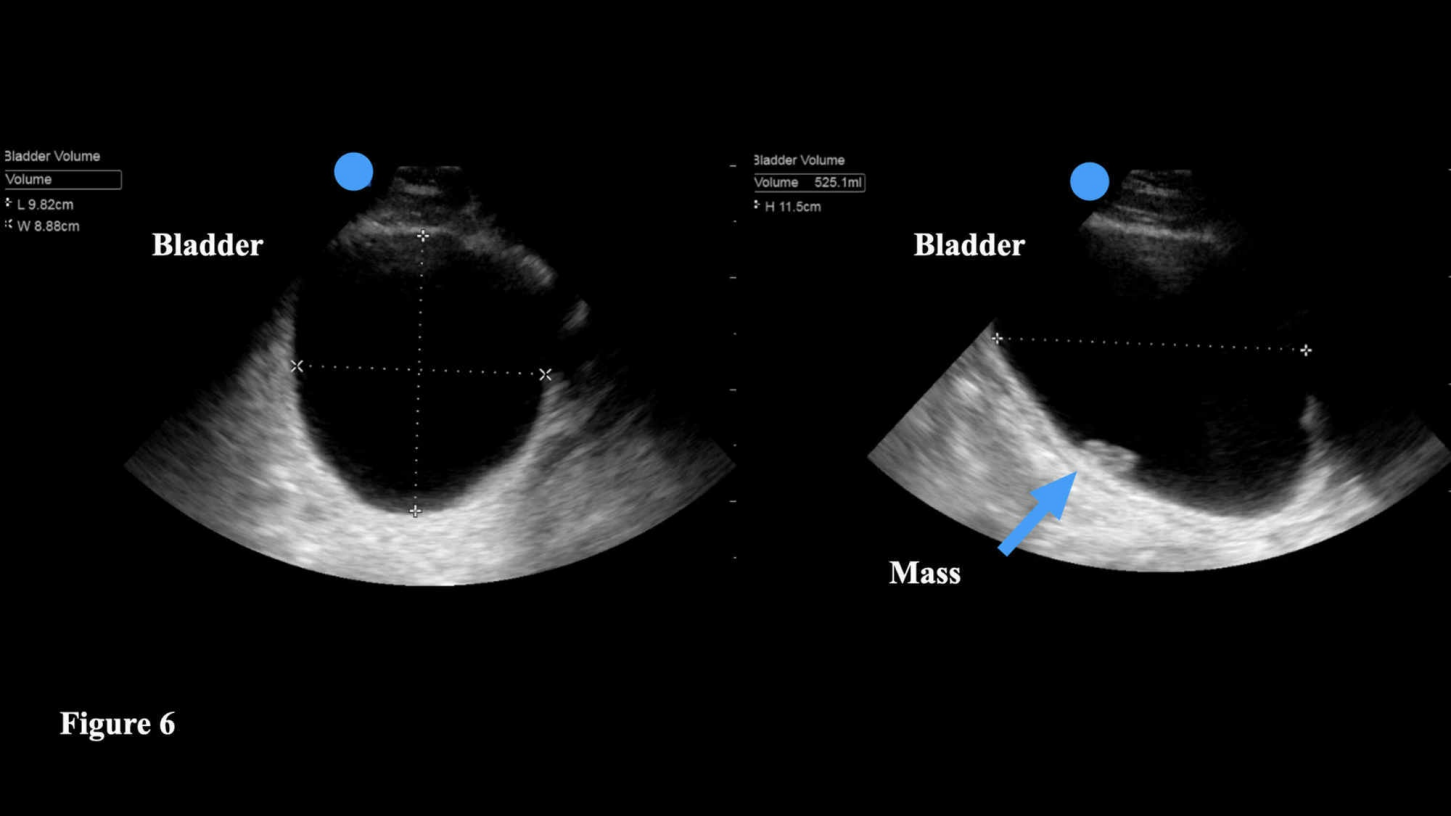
The height of the bladder (h) can be found by measuring the longest sagittal (longitudinal) plane of the bladder. By turning the transducer into a transverse view, the physician can measure the maximal length (l). In the same plane, measure the maximum width (w). The majority of ultrasound machines will automatically calculate the bladder volume when these three measurements are saved. This image also shows an incidentally found bladder mass (arrow).
